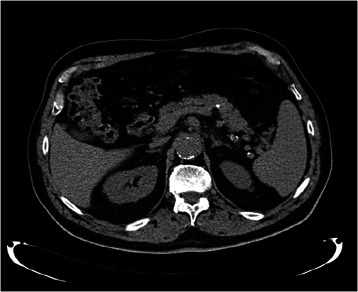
Abdominal CT native: normal pancreatic structure (after eight months).
